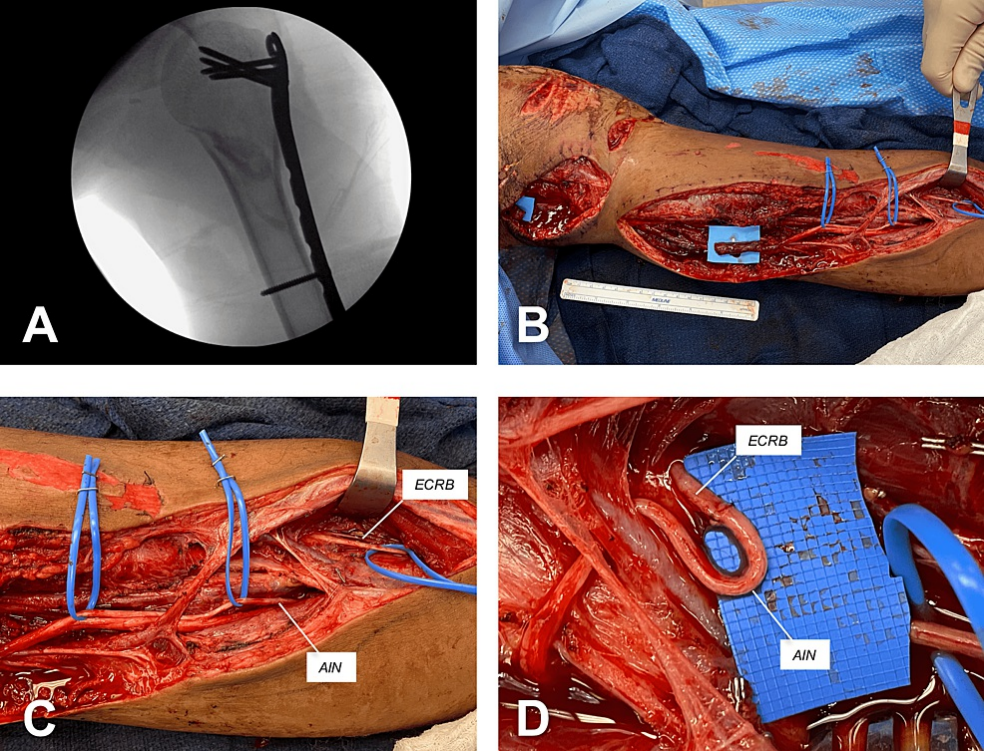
Initial surgical reconstruction of left upper extremity injuries. (A) X-ray showing bony stabilization with a proximal humerus locking plate. Interposition vein graft for brachial artery transection was also performed. (B) Median nerve transection with stumps nearly 15 cm apart. (C) Dissection of extensor carpi radialis brevis nerve (ECRB) and anterior interosseous nerve (AIN). (D) ECRB-to-AIN transfer by performing end-to-end coaptation with four simple interrupted stitches of 8-0 nylon. The coaptation site was subsequently wrapped with a nerve protector.
